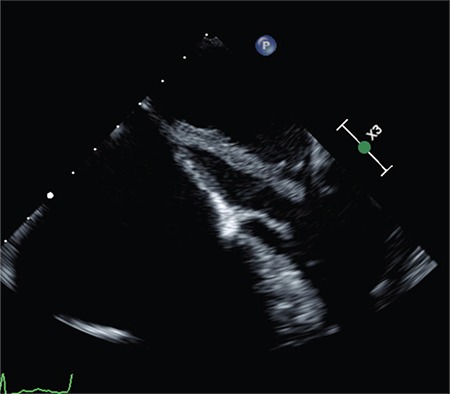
Transesophageal echocardiogram showing a mass of 4.3 cm originating from the left atrium and protruding into the mitral orifice.
